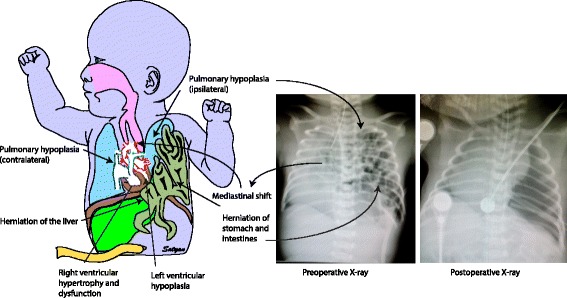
Anatomical and radiological features of CDH – A defect in the diaphragm causes the abdominal viscera to herniate into the thoracic cavity. Left sided hernias are common (85%) which results in herniation of both small and large intestines along with solid intra-abdominal organs. Herniation of viscera into the thoracic cavity results in abnormal lung development on the ipsilateral side with variable effect on the contralateral side. The effect of abnormal lung development on the contralateral side depends on the extent of herniation and the effect on mediastinal shift. Pulmonary hypoplasia results in abnormal pulmonary vasculature resulting in persistent pulmonary hypertension leading to right ventricular dysfunction. This is more pronounced after transitioning from fetal circulation. Left ventricular dysfunction can be secondary to direct compression in left sided hernia and secondary to low ventricular volumes in right sided hernias. Pre-operative chest and abdomen x-ray shows the air and fluid filled loops of bowels on the left side of the thorax with the endotracheal tube above the thoracic vertebra level 4 pushed towards the right side signifying mediastinal shift. (*Copyright Satyan Lakshminrusimha)*
